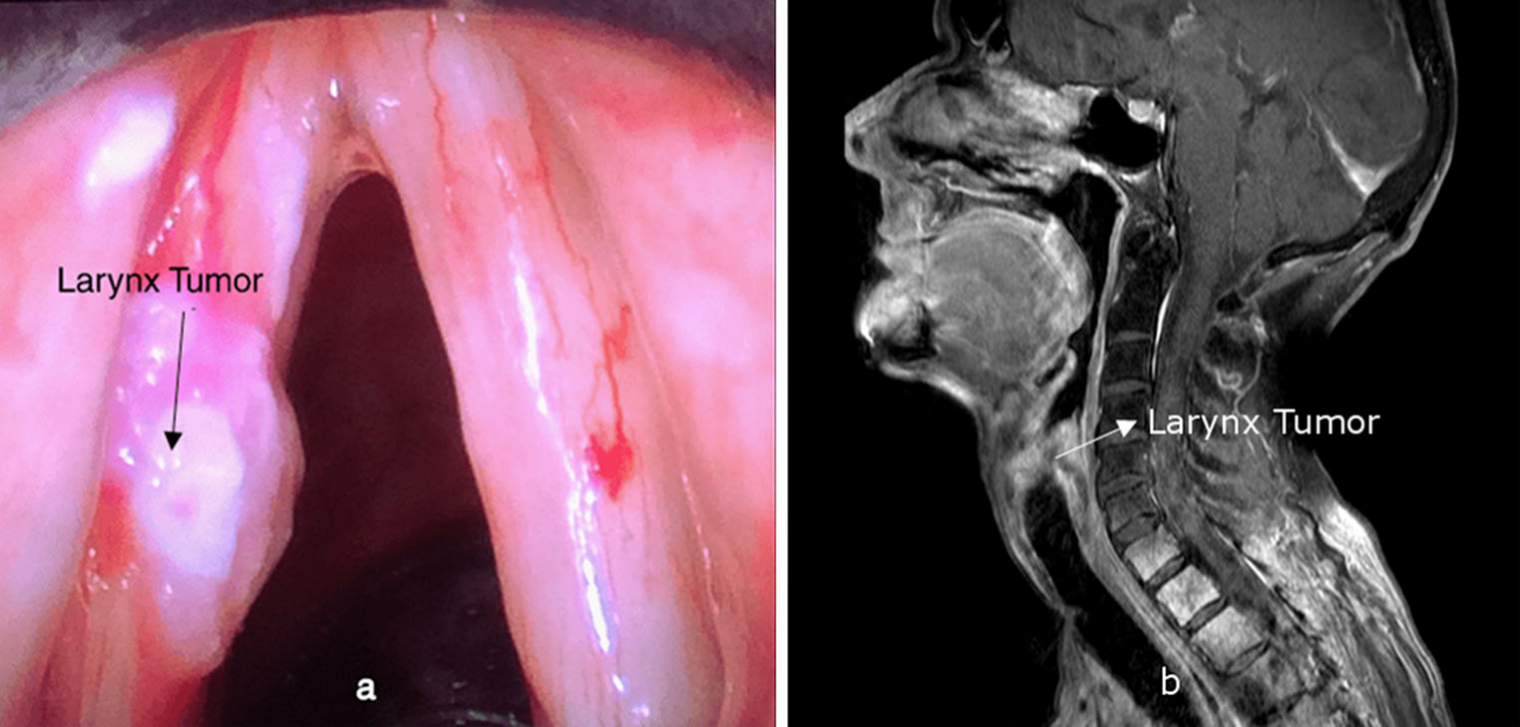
a Endoscopic image of a tumour on the vocal fold. b MRI Image of a patient showing the larynx tumour (Images: Department of ENT, OVGU Magdeburg)
